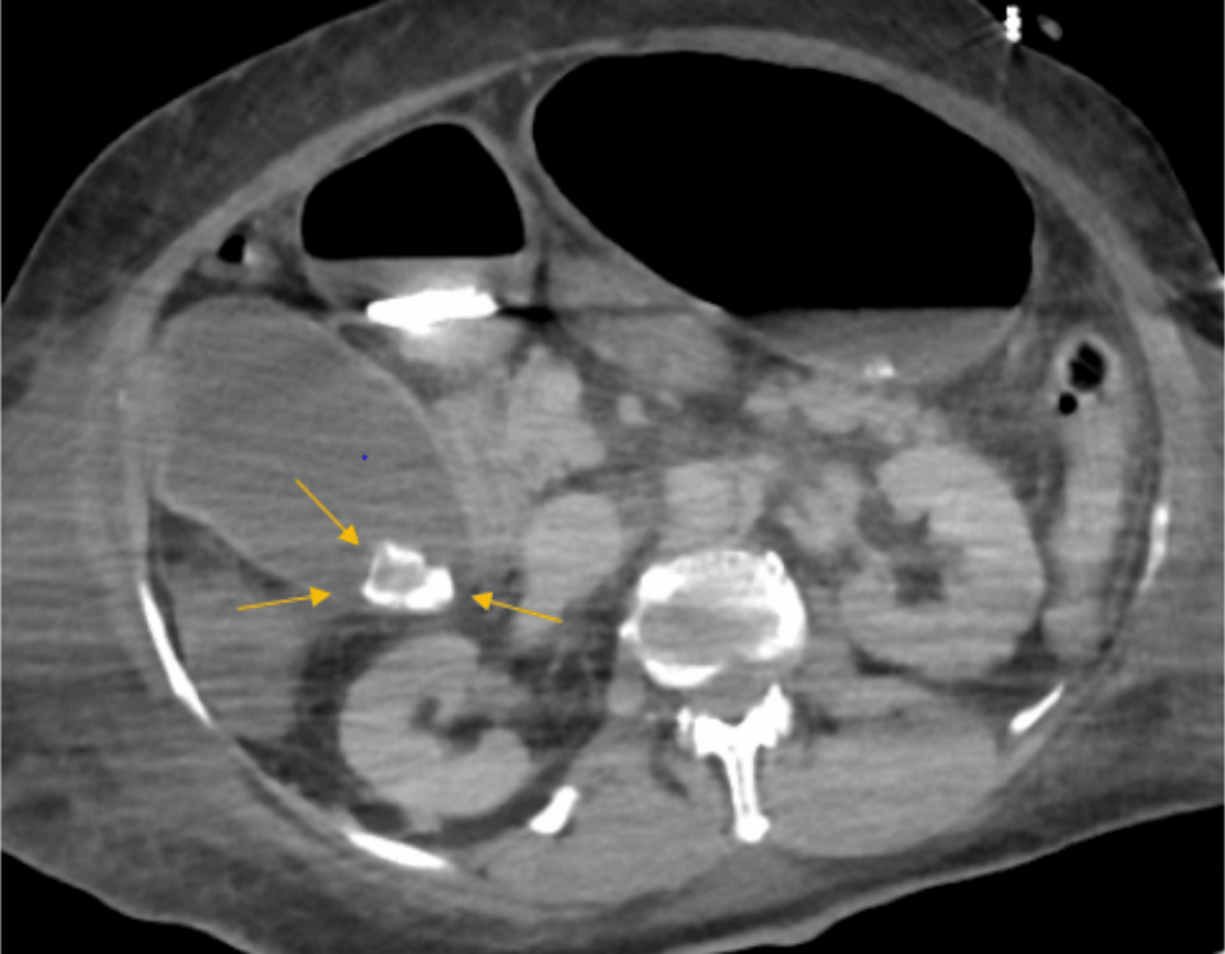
Computed tomography (CT) scan of the abdomen. Distended gallbladder with multiple gallstones with wall thickening, indicative of acute calculus cholecystitis.
